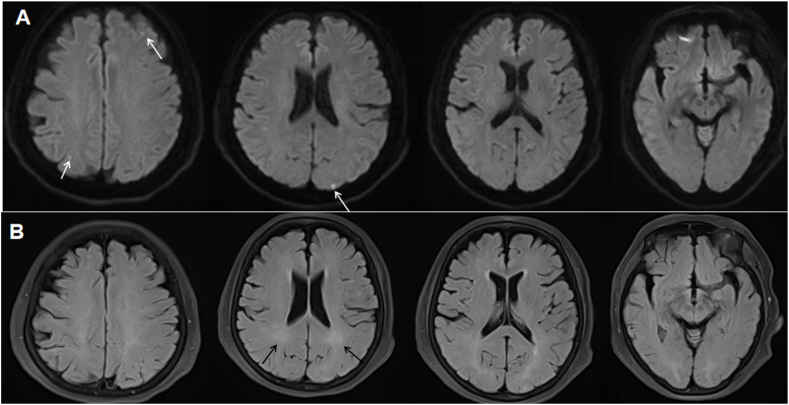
MRI findings at coma status following a hypoxic event induced by poppy overdose. DWI showed acute multifocal punctate infarcts (white arrows) (A) and FLAIR sequence demonstrated mild white matter demyelination (black arrows) (B). MRI = magnetic resonance imaging; DWI = diffusion weighted imaging; FLAIR = fluid-attenuated inversion recovery.
